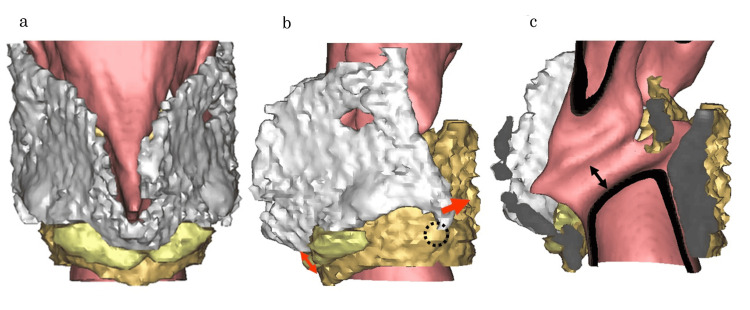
3D CT results of Case 1. (a) Frontal view. (b) Lateral view. (c) Lumen. White: thyroid cartilage; bronze: cricoid cartilage; yellow: Gore-Tex® (WL Gore and Associates, Inc., Flagstaff, US). The cricoid and thyroid cartilages were overlapped, with the cricoid cartilage entering the thyroid cartilage (red two headed arrow). The inferior horn of the thyroid cartilage was deviated considerably posterior to the cricothyroid joint (red arrow). The vocal cords had not lost thickness (black two headed arrow). 3D CT: Three-dimensional computed tomography
